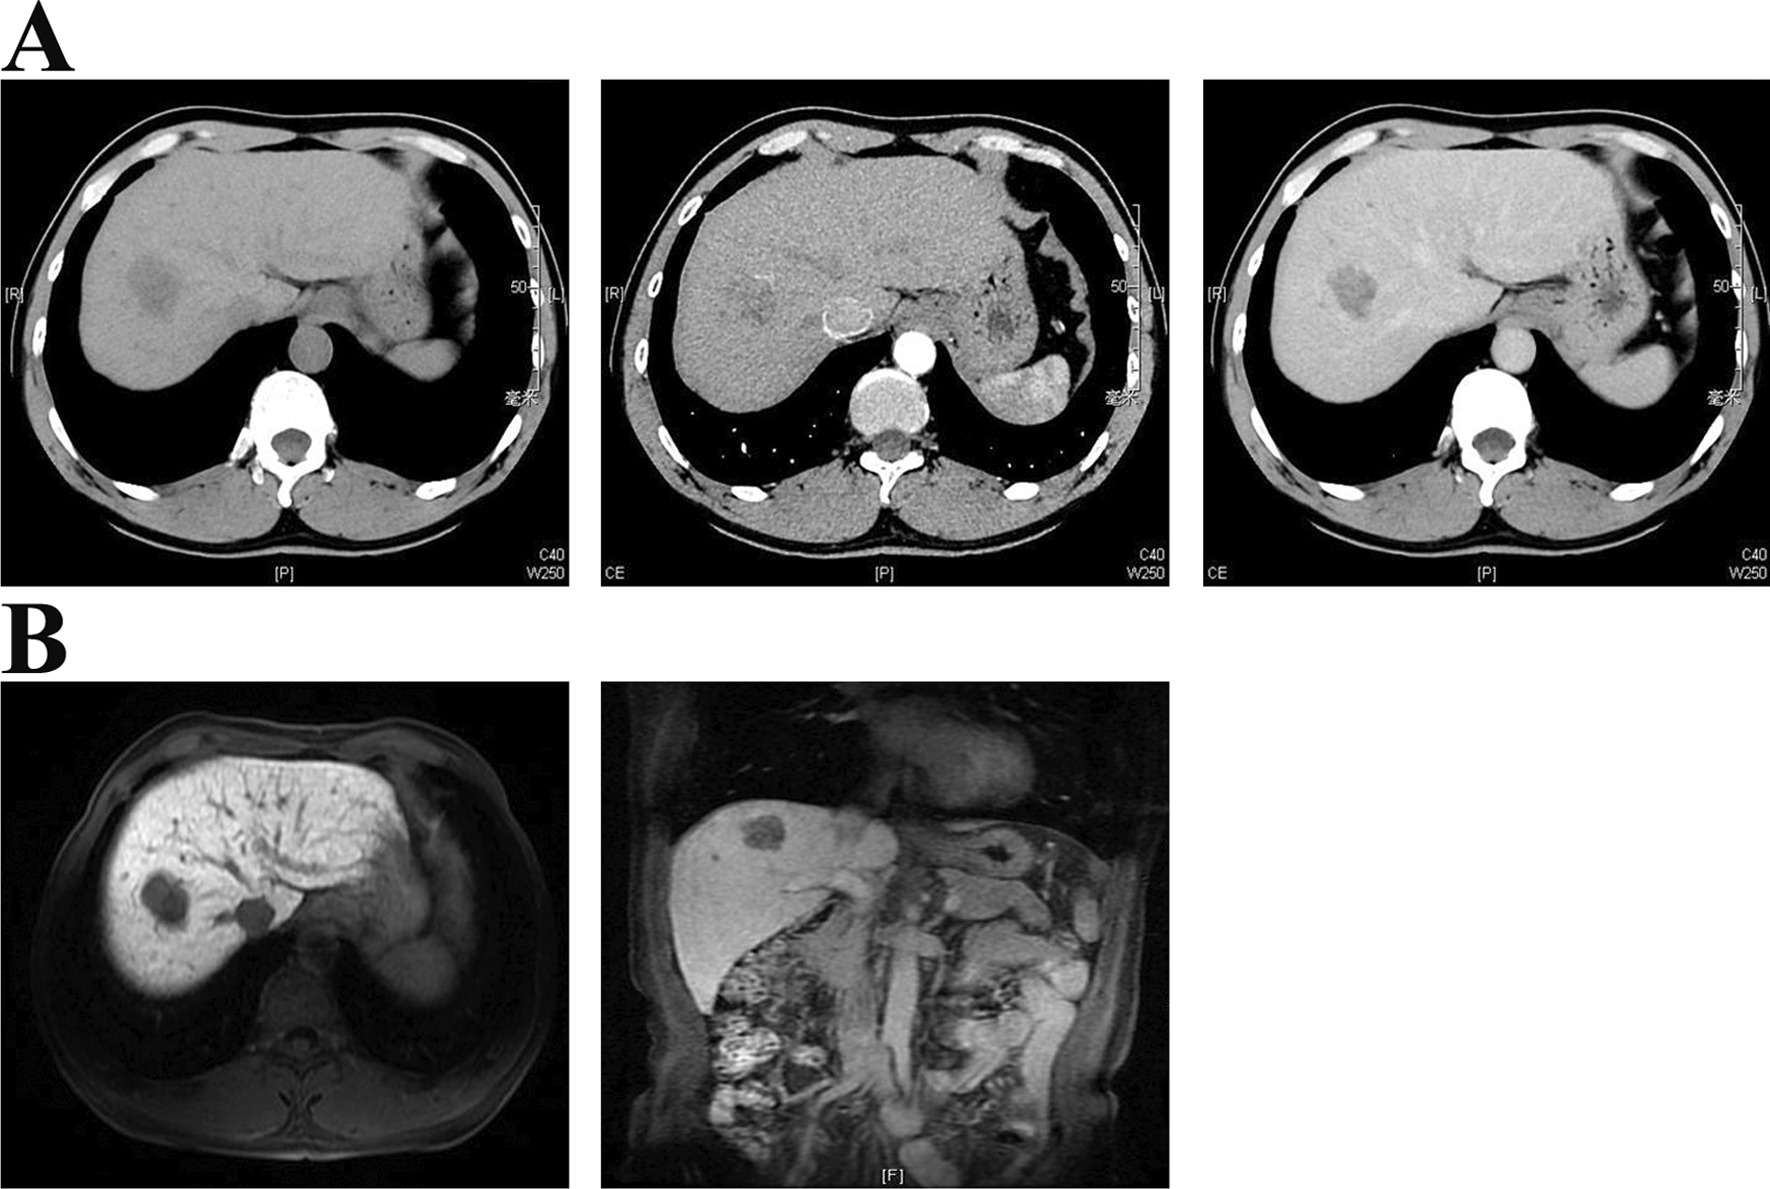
Preoperative CT (a) and MRI (b) of the liver. A 3.2 cm × 3.0 cm sized mass (arrows) was found on S8
